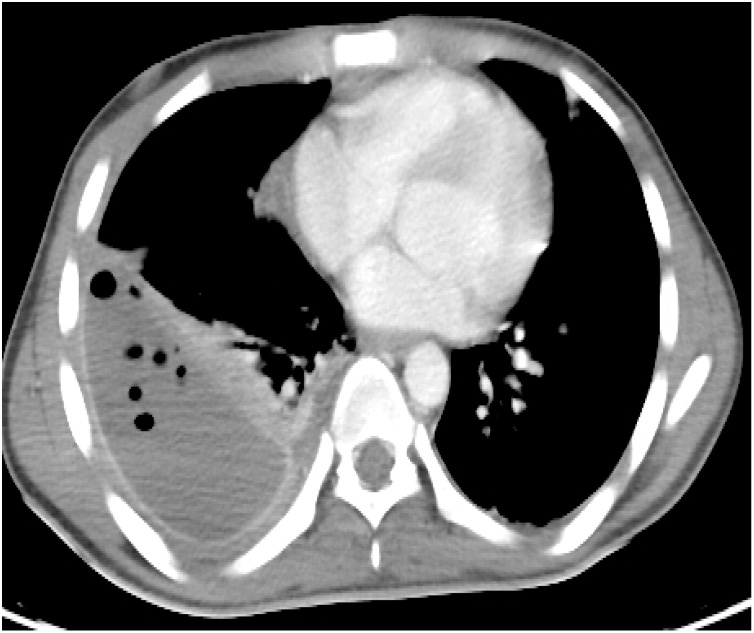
CT Chest shows the well-formed right sided empyema.
